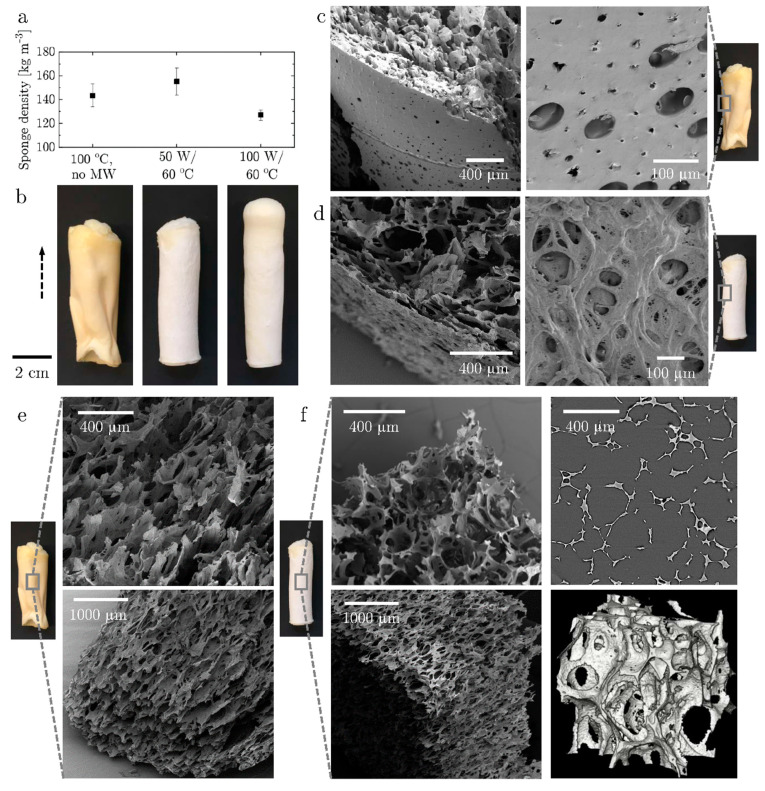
Macro- and microstructure of the protein sponge dried with and without superposition of microwave heating: (a) average densities of dried protein sponges at different drying conditions; (b) macroscopic images of dried protein sponges at different drying conditions (the dashed arrow indicates the expansion direction); (c) surface layer formed after drying at 100 °C without microwave heating; (d) surface layer of protein sponge dried at 50 W/60 °C; (e) inner structure formed after drying at 100 °C without microwave heating; (f) inner structure of protein sponge dried at 50 W/60 °C investigated by SEM (left) and μ-CT (right). The corresponding SEM images of the protein sponge dried at 100 W/60 °C are found in the Supporting Information Figure A (Supplementary Materials).
